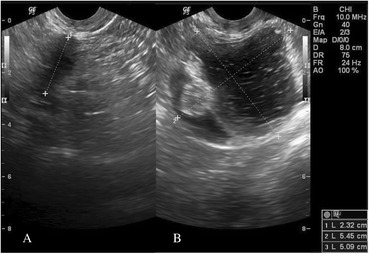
a Transvaginal ultrasonography of the right ovary shows no abnormal findings. b Transvaginal ultrasonography shows a left ovarian mass containing the solid portion representing a hyperechoic lesion and the fluid portion representing a hypoechoic lesion
